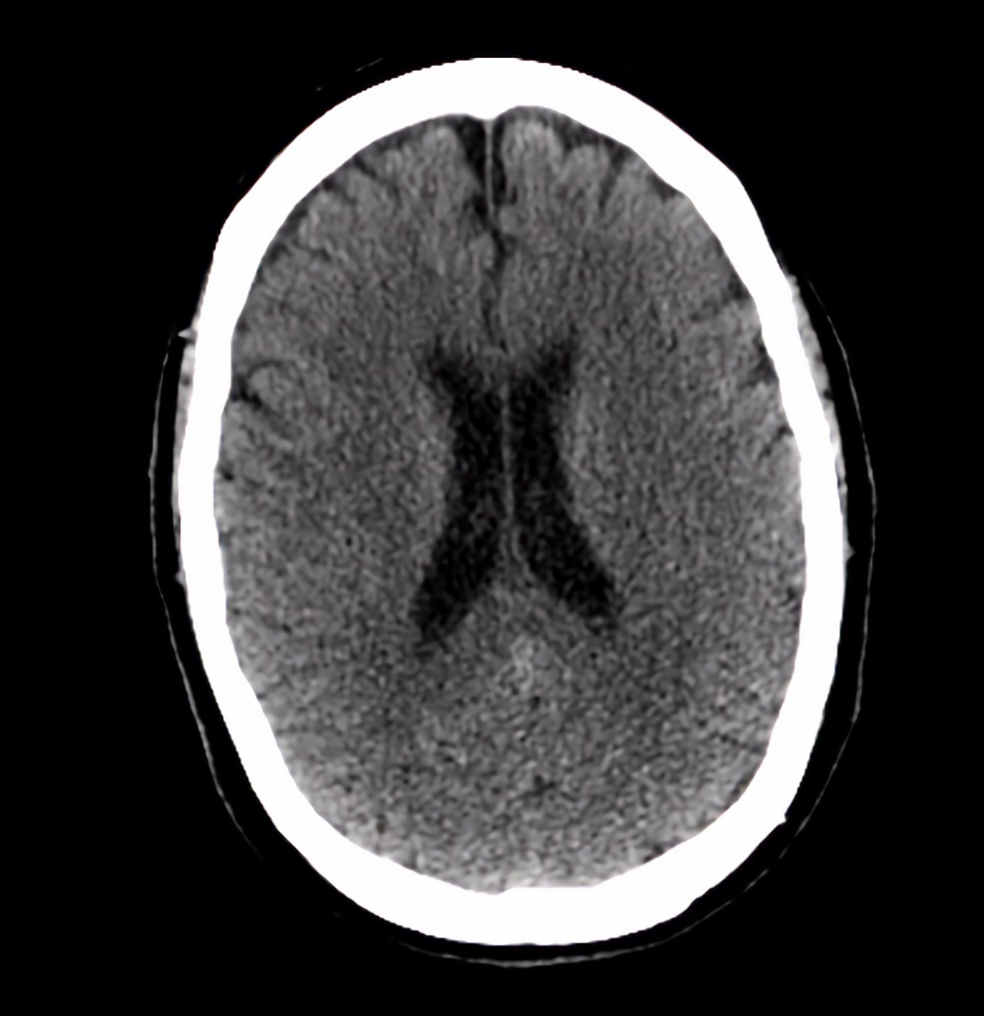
CT brain without contrast. No acute intracranial bleed, mass, or midline shift was noted.
